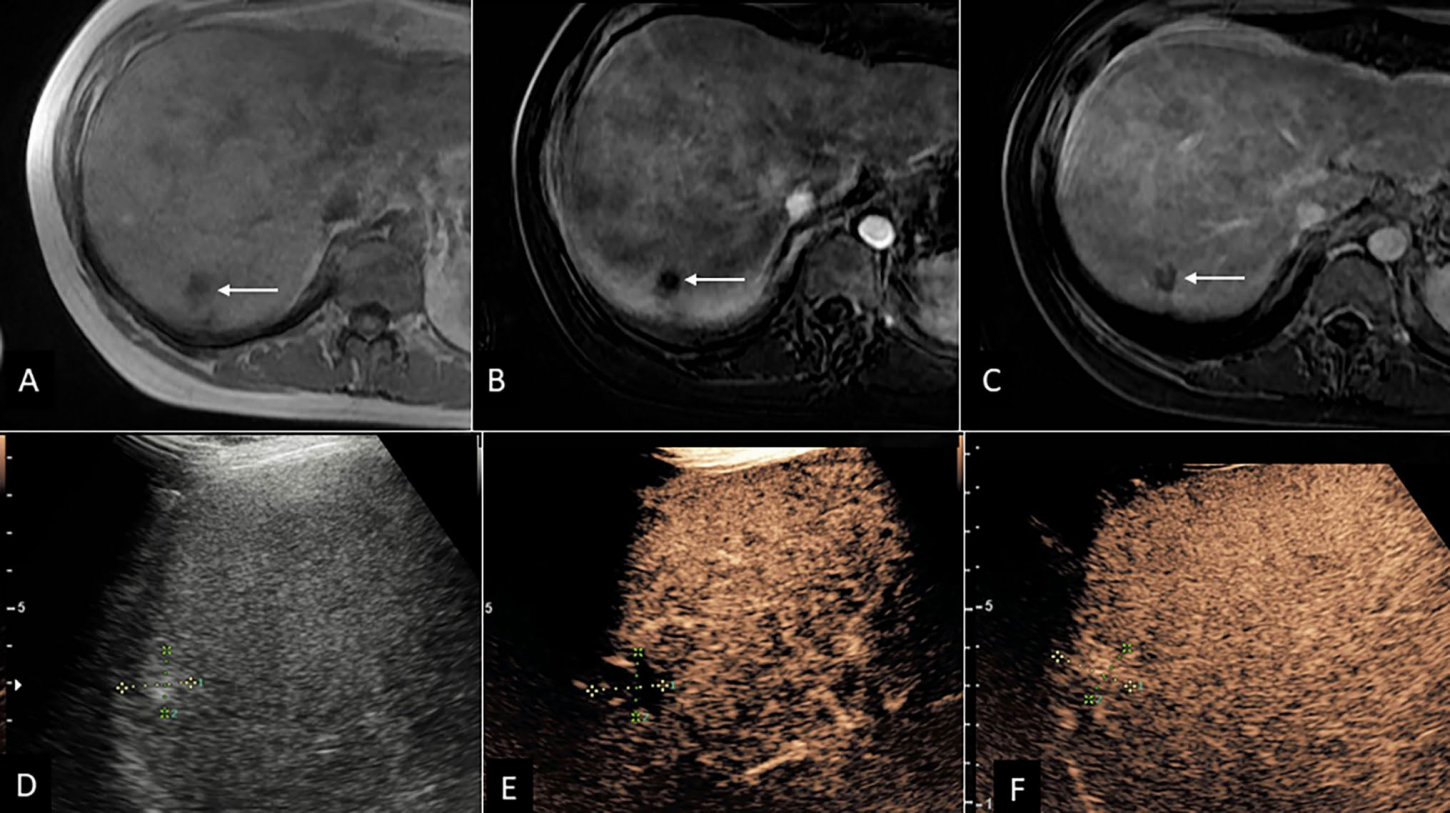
Hemangioma with MRI LR-3 categorization. A Pre-contrast MR image demonstrating small hypo-intense observation in the right hepatic dome (arrow). Arterial phase image which is degraded by motion artifact (B) demonstrated questionable arterial hypo enhancement in the parts of the observation, with hypoenhancement on late phase image (C), resulting in LR-3 categorization. D Grayscale ultrasound image showing hyperechoic liver nodule measuring 1.6 cm marked by calipers with peripheral interrupted APHE (E). CEUS representative image at 1 min (F) persistent hyperenhancement—typical appearance of hemangioma (LR-1)
